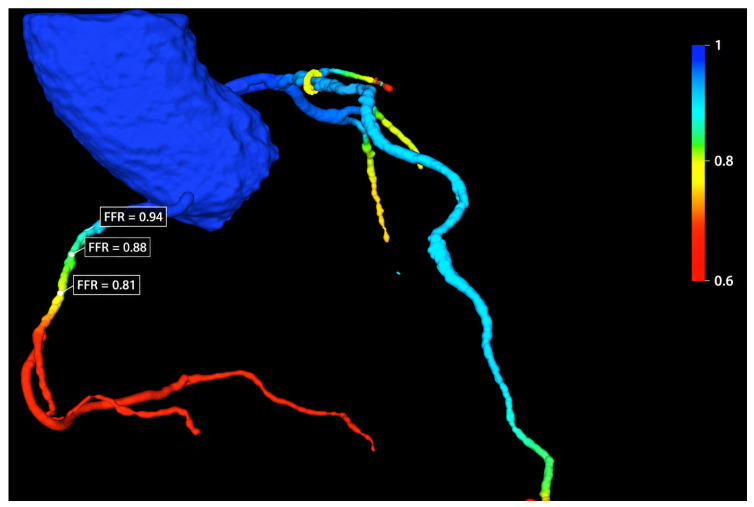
A 75-year-old female with a proximal LAD stent (Segment 6). Physiological post-stent CT-FFR (0.92; Delta FFR 0.03) was achieved while pathological CT-FFR in the RCA (70–99% stenosis, CAD-RADS 4A) preceded before posterior-wall MI. LAD remained free of significant stenosis on ICA.
